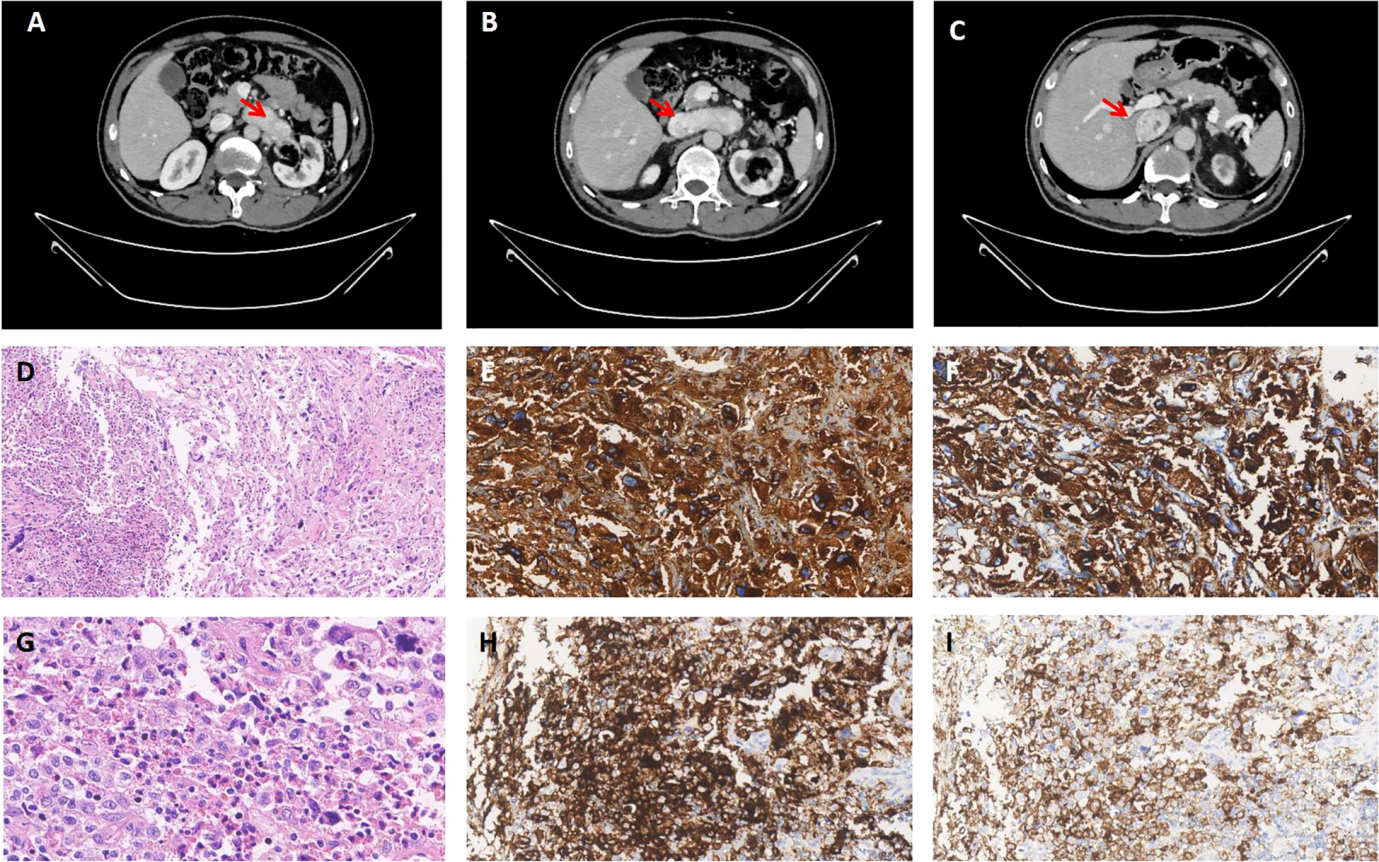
Computed tomographic angiography and pathological images. (A-C) The lumen of the left renal vein showed a soft tissue mass extending from its junction with the inferior vena cava, along the course of the inferior vena cava. The enhanced scan exhibited significant enhancement. Pheochromocytoma in metastasis. (D) The mononuclear cell area on the left side exhibits a significant presence of numerous eosinophils(x100 HE). (E, F) Pheochromocytoma expressing CgA and syn (x200). (G) The monocytic cells have reniform-to-oval nuclei and a central horizontal groove(x400 HE). (H, I) The mononuclear cells are positive for CD1a and Langerin (x200).
